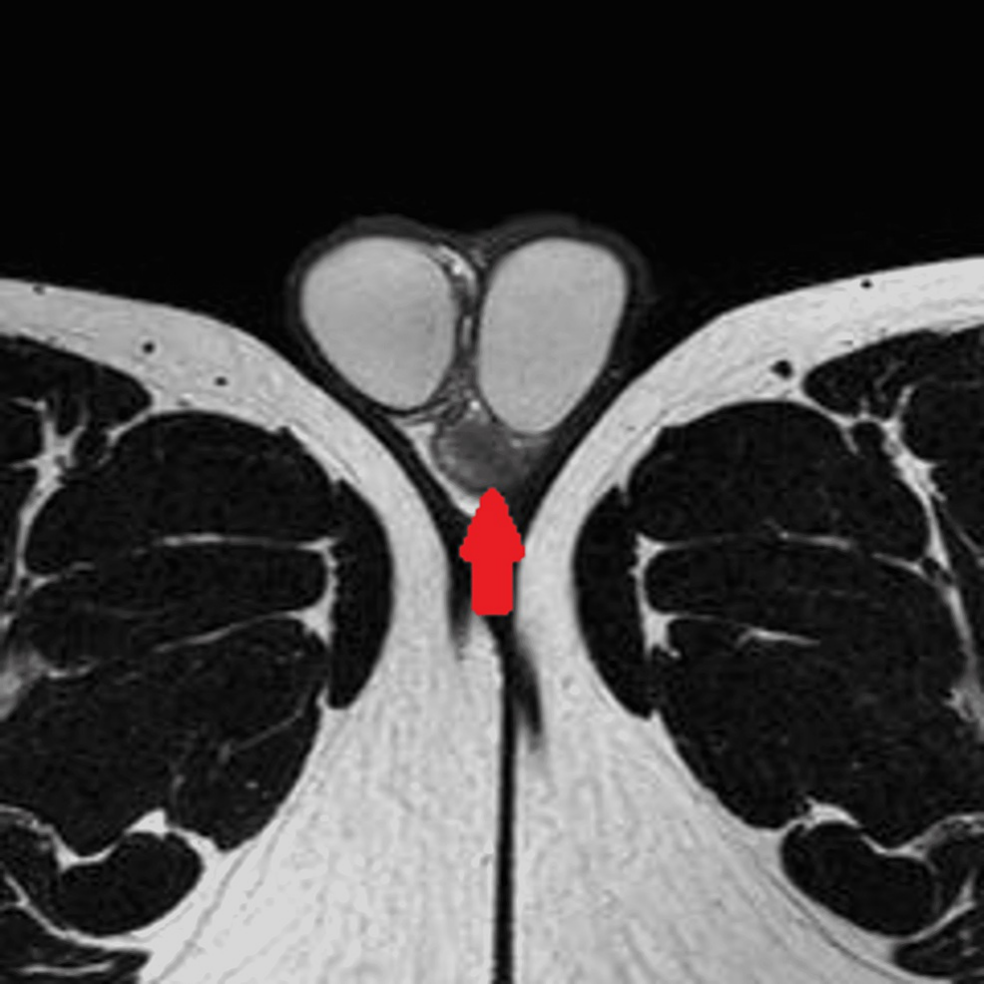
Scrotal MRI Scrotal MRI revealed a 3×1.9 cm extratesticular mass in the tail of the left epididymis. The mass demonstrates dark signal intensity on T1- and T2-weighted sequences. It is separable from the testicle and not attached to the scrotal wall. MRI, magnetic resonance imaging.
